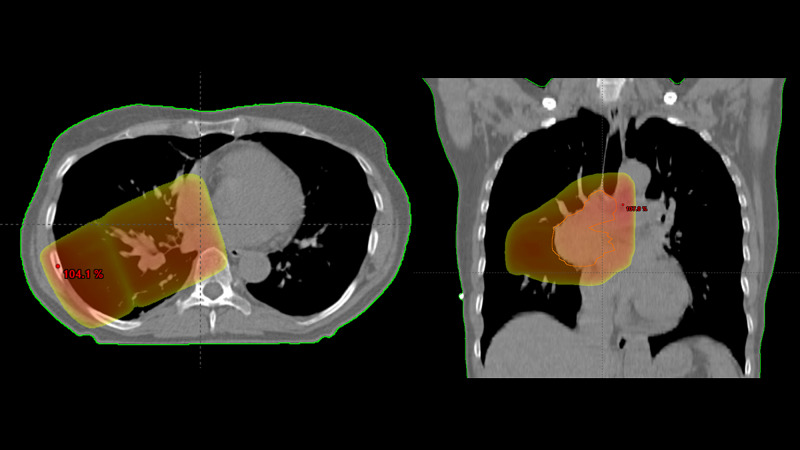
Representative axial and coronal treatment planning computed tomography scan showing the 80% isodose color wash in a patient treated for right-sided T2 N2 disease (15 fractions of 2.8 Gy) who was hospitalized for cardiac arrhythmia The maximum dose is displayed in red. The orange line depicts the clinical target volume.
